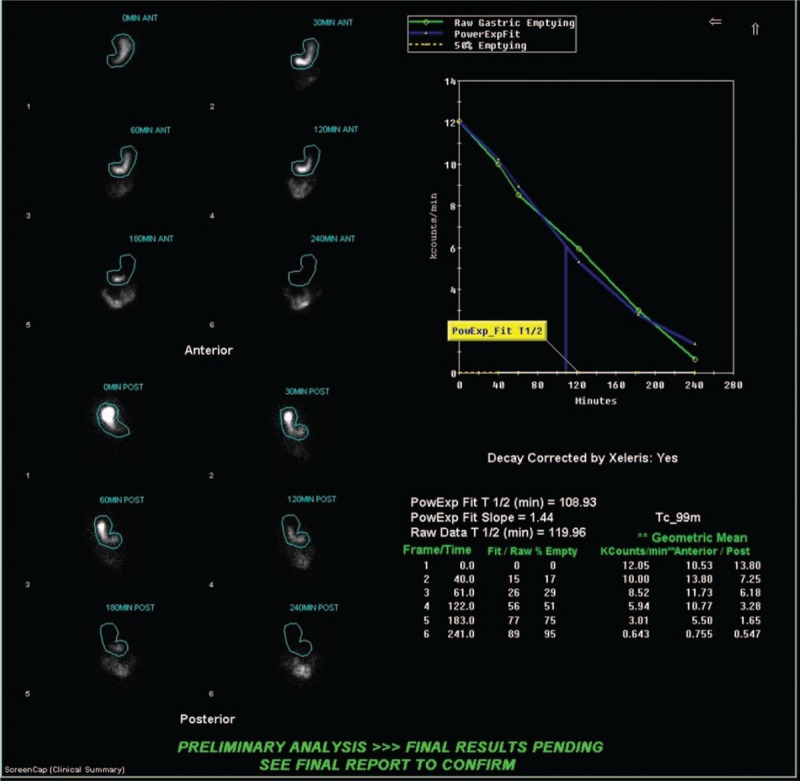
Normal gastric emptying scintigraphy in a 58-year-old woman who underwent LSG. Pre-op GES: normal fundic accommodation; at 2 h: 49%, N < 60% and at 4 h: 5%, N < 10%. Patient had a normal postoperative outcome.
